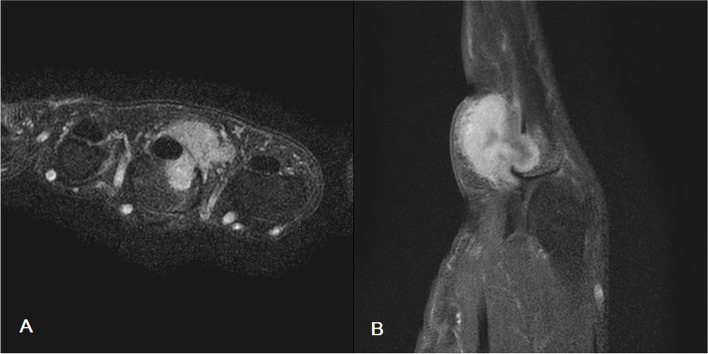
MRI of the finger confirmed the presence of a mass (1.9 × 1.8 × 1.9 cm) encircling the third flexor digitorum tendon, and bony invasion was observed. A Axial view (T2-weighted image). B Sagittal view (T1-weighted, fat suppressed image)
